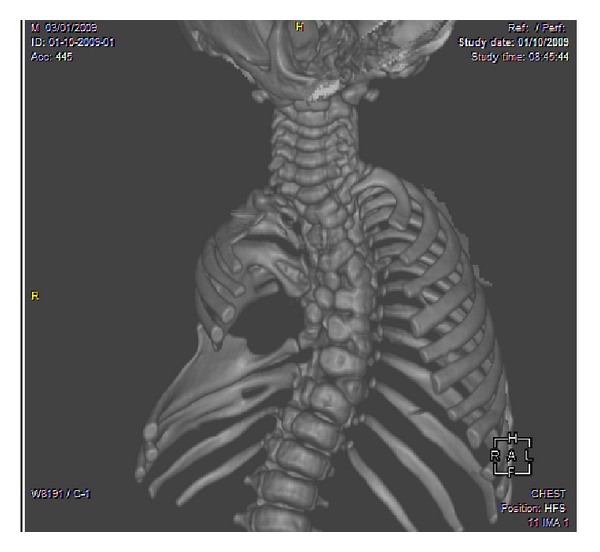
Three-dimensional CT scan AP thoracic image revealing full details of block, fused, and hemivertebrae as well as misaligned, absent, fused, and hypoplastic ribs at the right side and supernumerary ribs at the opposite side.
